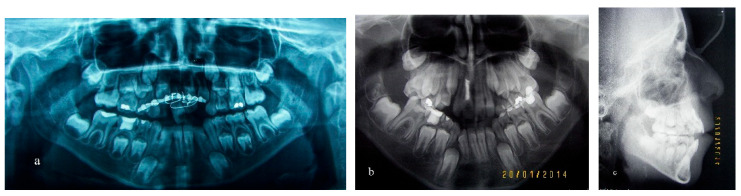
Radiographic examination. (a) Panoramic radiograph after repositioning and splinting, (b) panoramic radiograph after endodontic treatment, (c) lateral cephalogram before orthodontic treatment.
